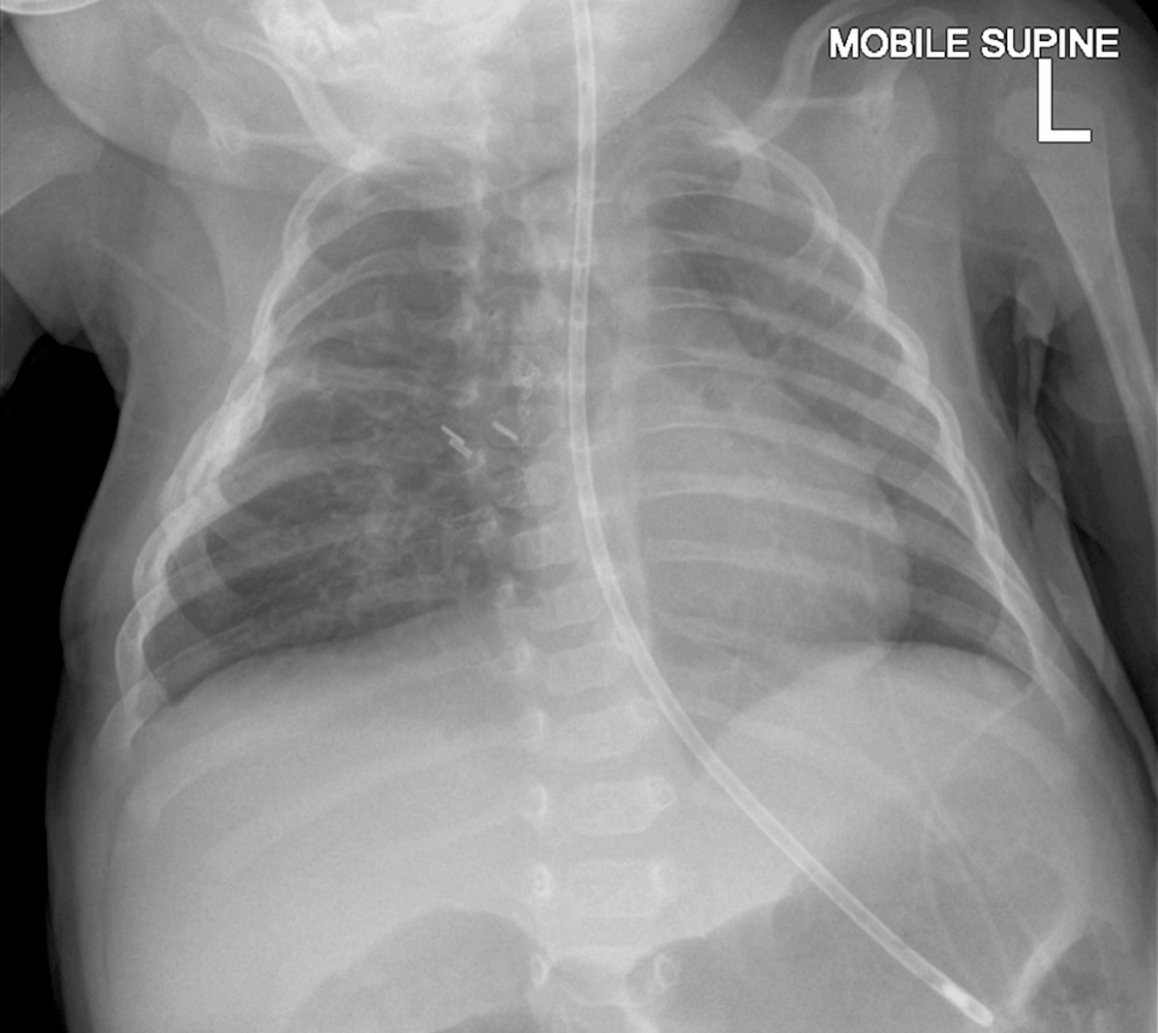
Postoperative chest X-ray The chest deformity at the site of the thoracotomy remains stable in appearance. The lungs are well-aerated, and the cardiomediastinal contours are normal.
